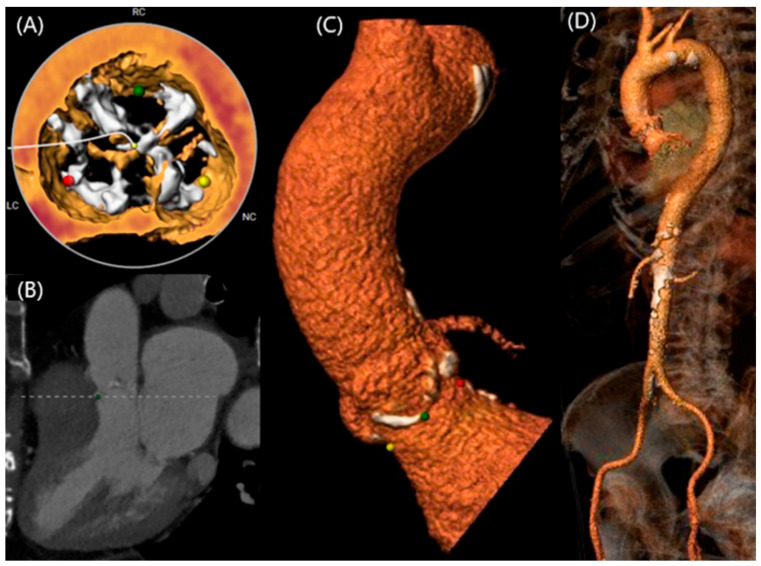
Female 93-year-old patient with severe BAV stenosis who underwent TAVI. Case from the Cath lab from “Hippokration” General Hospital Athens. Images from MDCT during preoperative planning demonstrating: (A) anatomy of the BAV and its degree of severity, (B) double oblique projection with CT reconstruction for the evaluation of the aortic annulus and ascending aorta, (C) 3D reconstruction of the aortic root and ascending aorta, (D) 3D reconstruction of the aortic root ascending aorta, aortic arch, descending aorta, and iliac arteries. The three color dots at the figure represent the 3D points for the visualization of the annular base.
